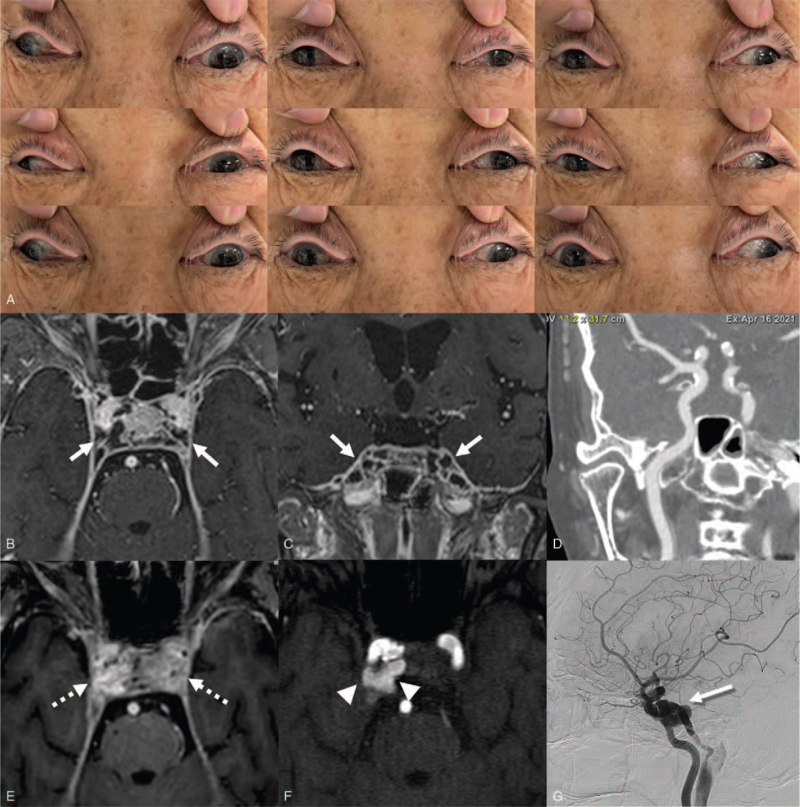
Clinical presentations and neuroimages of the patient. The 9 cardinal gaze photographs show incomplete ptosis, eyelid swelling, and exotropia associated with medial and vertical gaze limitations in both eyes (A). Initial gadolinium-enhanced brain MRI reveals thrombotic filling defects in both cavernous sinuses (B, C, arrows). However, the evidence of fistula or aneurysm involving the internal carotid artery is absent on initial neck computed tomography angiography (D). Follow-up gadolinium-enhanced brain MRI shows remnant thrombotic filling defects and enlarged cavernous sinuses with prominent contrast enhancement (E, dotted arrows). Also, Time-of-Flight MR angiography shows increased flow-related signal within right cavernous sinus suggesting carotid-cavernous fistula located at a distal cavernous segment of the right internal carotid artery (F, arrowheads), which is confirmed by digital subtraction angiography (G, shadowed arrow).
